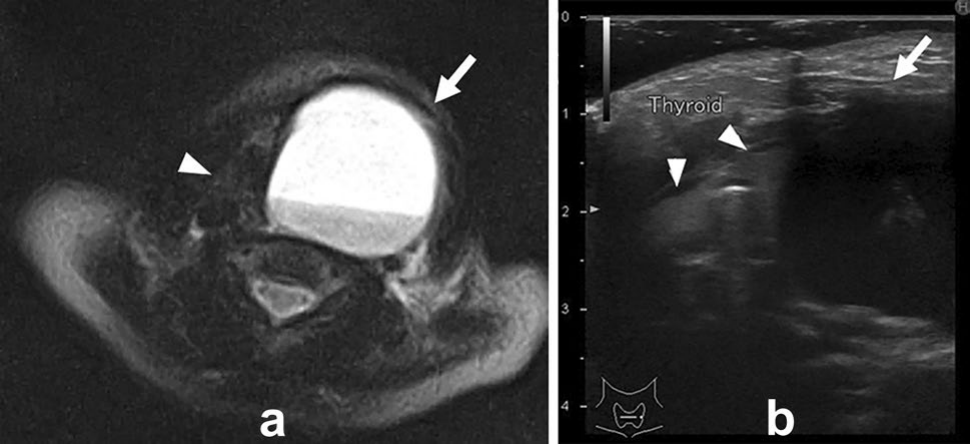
Ten-day-old girl with pyriform fistula (forth brachial cleft cyst) T2 weighted image a and ultrasonography b showed a cystic lesion (arrows) on the lateral side of the left lobe of the thyroid (arrow heads)
